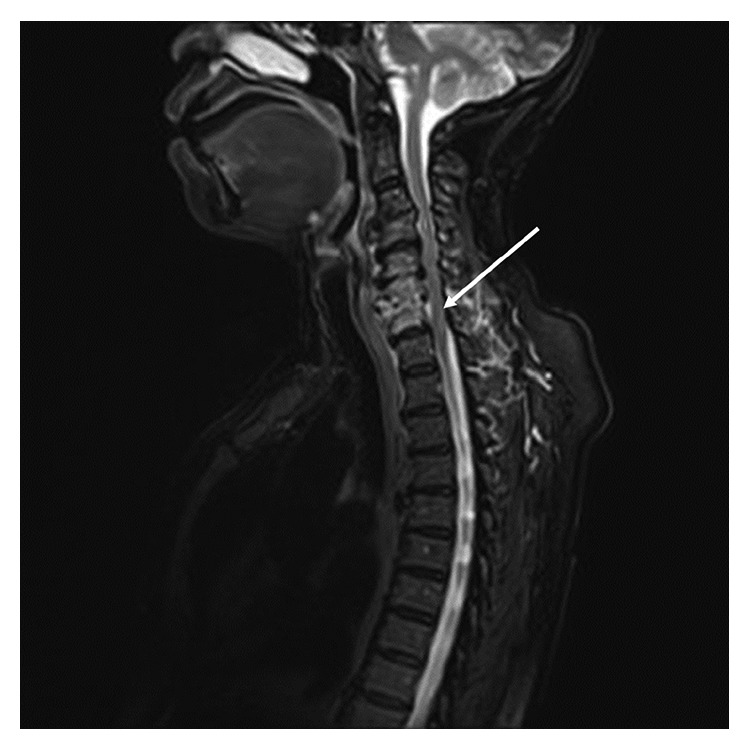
MRI of cervical spine demonstrating epidural abscess from MRSA.
